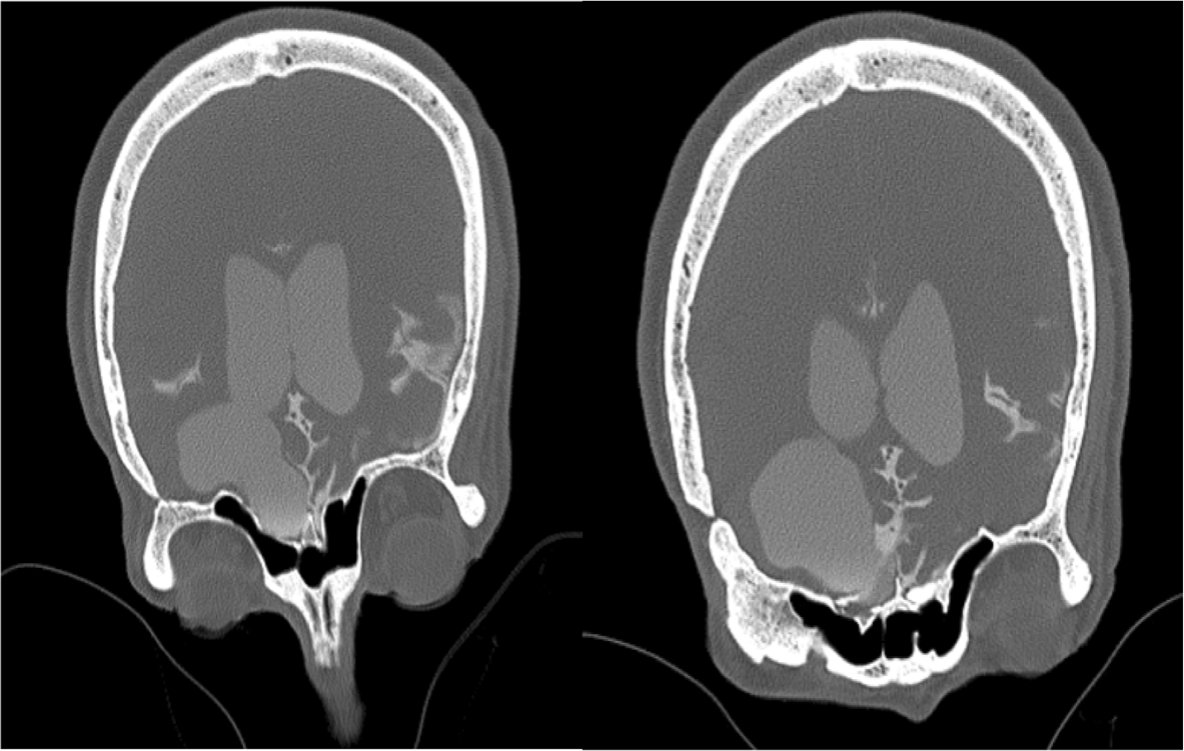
CT cisternography showing no contrast leak into paranasal sinuses
